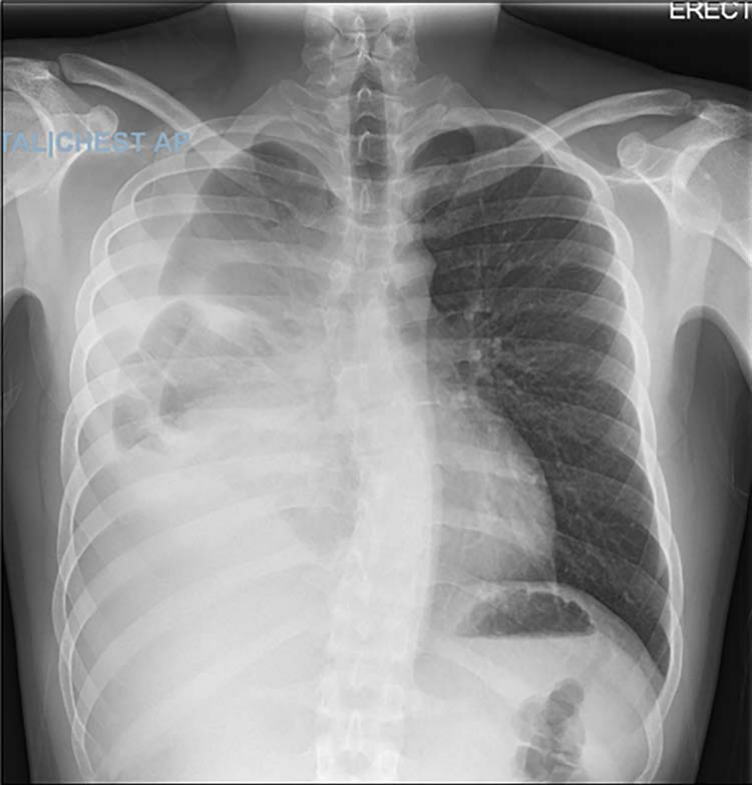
Chest X-ray demonstrating large right pleural effusion.
